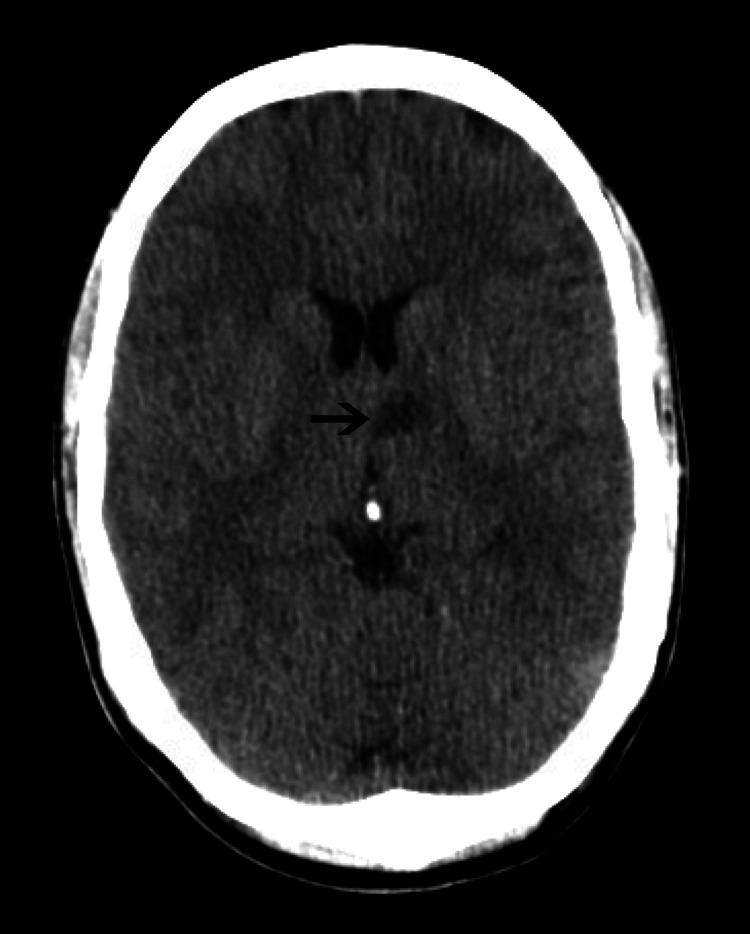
CT of the head showing subacute infarct on the left thalamus
